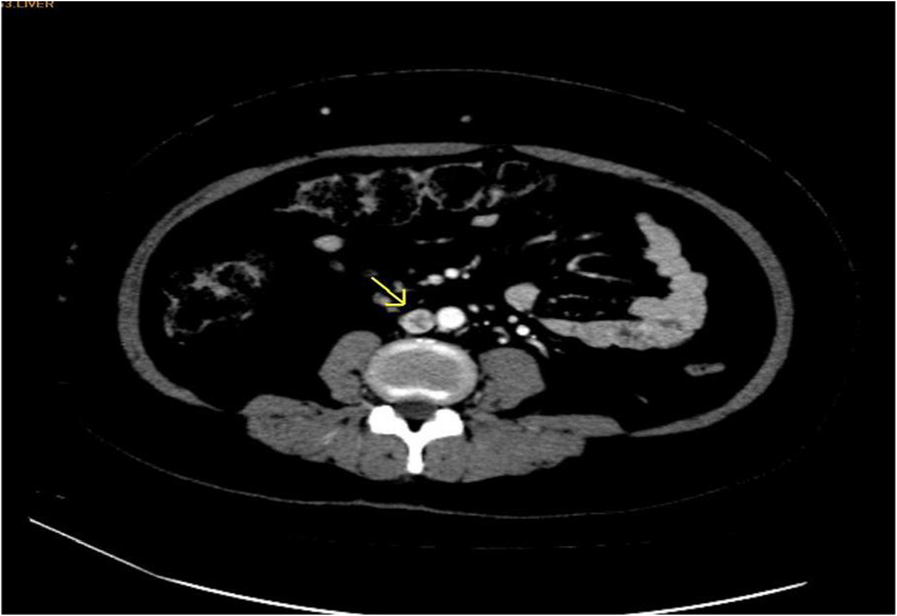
Computed tomography scan at the level of the renal hilum showing narrowing and thrombosis of the inferior vena cava (arrow).
